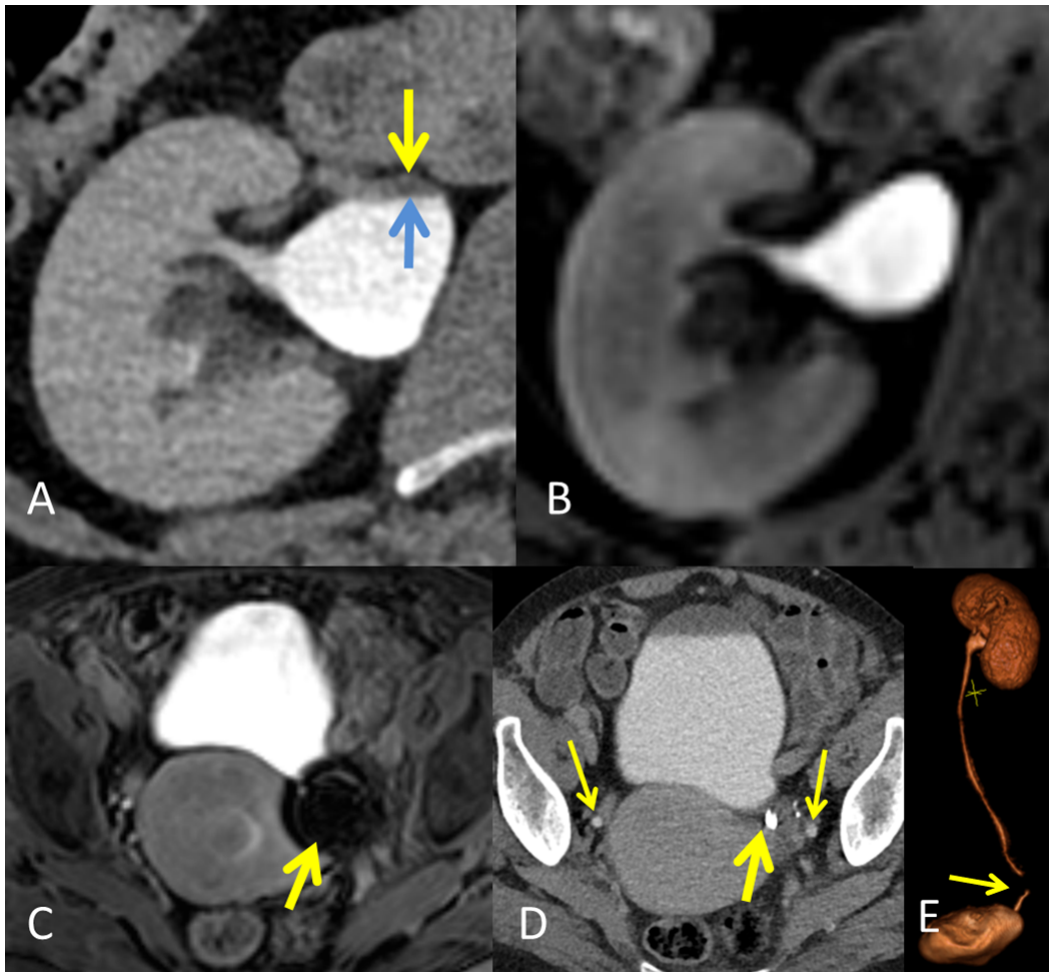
Artefacts encountered during imaging. The hydration protocol incorporated into the computed tomography resulted in better dilatation of the renal cavities as seen in image A (axial CT) compared to magnetic resonance excretory urography (MRU, image B), but occasionally at the expense of a contrast layering effect (Area between arrows). A susceptibility artefact due to the presence of a metallic sterilization clip in the MRU (image C, arrow) results in a void signal area. The clip produced no artefacts at CT (image D, thick arrow) and thin arrows show the position of distal ureters. The artefact at MRU impaired the visibility of a short ureteral segment as seen in the volume reconstruction MRU image E (arrow).
